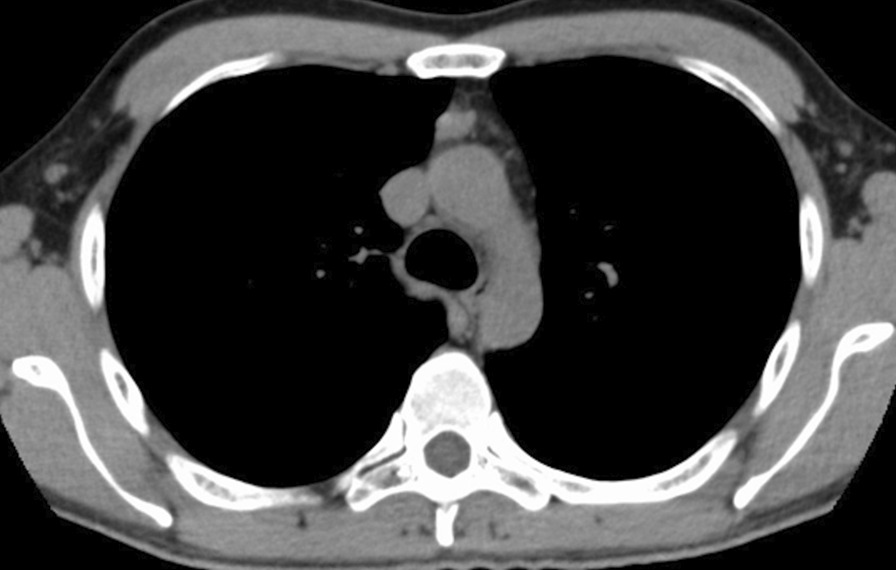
Computed tomography imaging. Chest computed tomography revealed an isolated anterior mediastinal mass with a maximum diameter measuring 22 mm without invasion to the surrounding tissues
